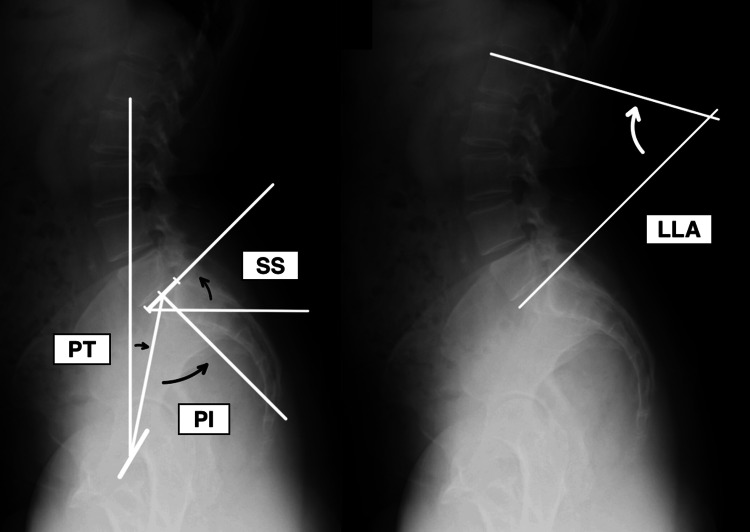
X-ray image of the hip joint while standing, sagittal plane SS: sacral slope; PT: pelvic tilt; PI: pelvic incidence; LLA: lumbar lordosis angle
